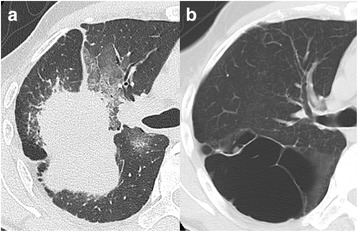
a A solitary mass in the right upper lobe of the lung. The mass was well-circumscribed and surrounded by a reticular shadow. The average HU value of the mass on HRCT was 35. b An emphysematous giant bulla of the lung was observed 1 year before the hemorrhagic presentation of the tumor
